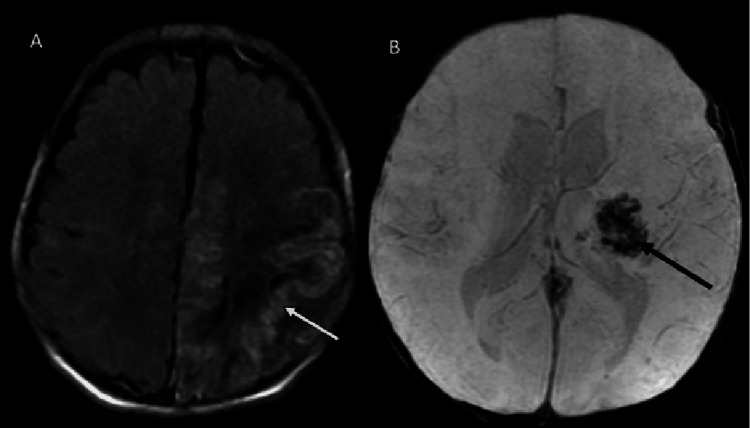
Axial fluid-attenuated inversion recovery (FLAIR) MRI image (A) on the follow-up MRI performed after 10 days shows laminar necrosis (arrow) and encephalomalacia in left frontal lobe. Susceptibility-weighted axial MRI image (B) shows hemosiderin from hemorrhage (arrow) in the left thalamus.
